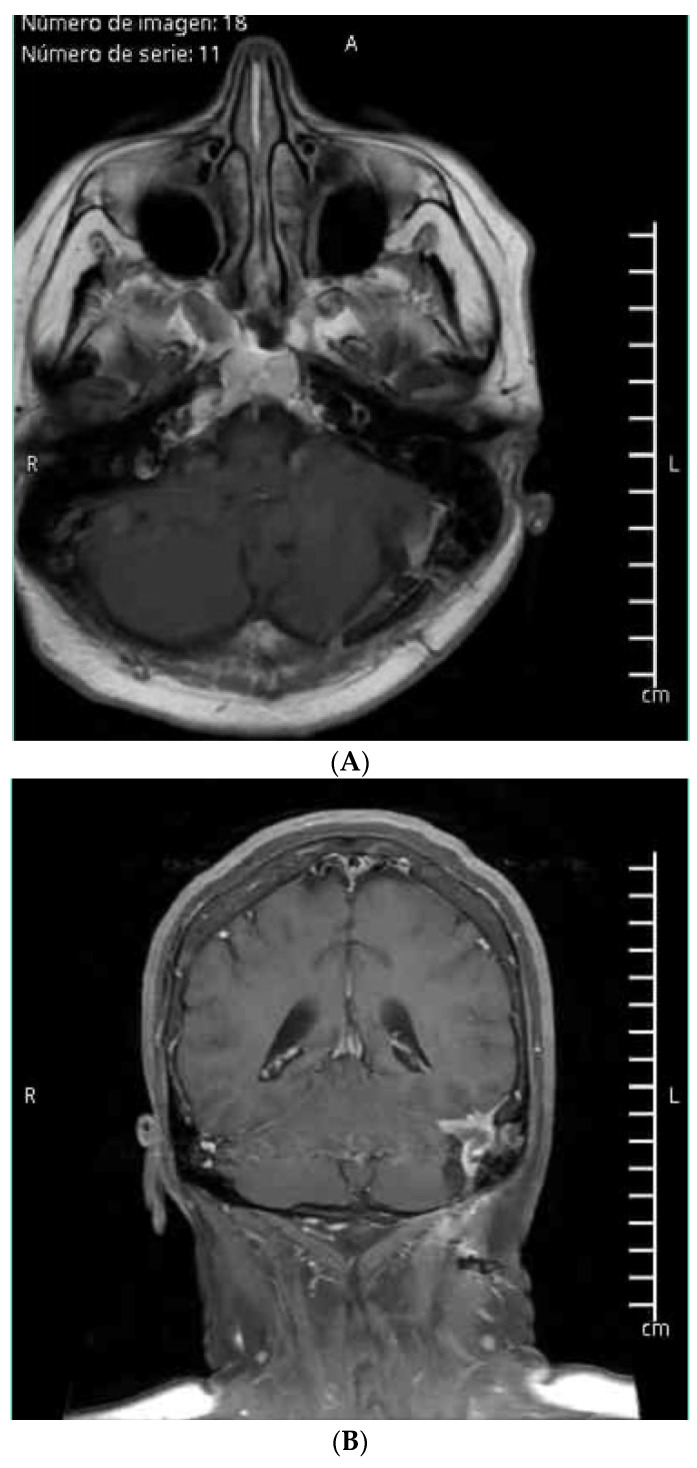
(A) MRI axial view post-procedure. (B) MRI coronal view post-surgery.
